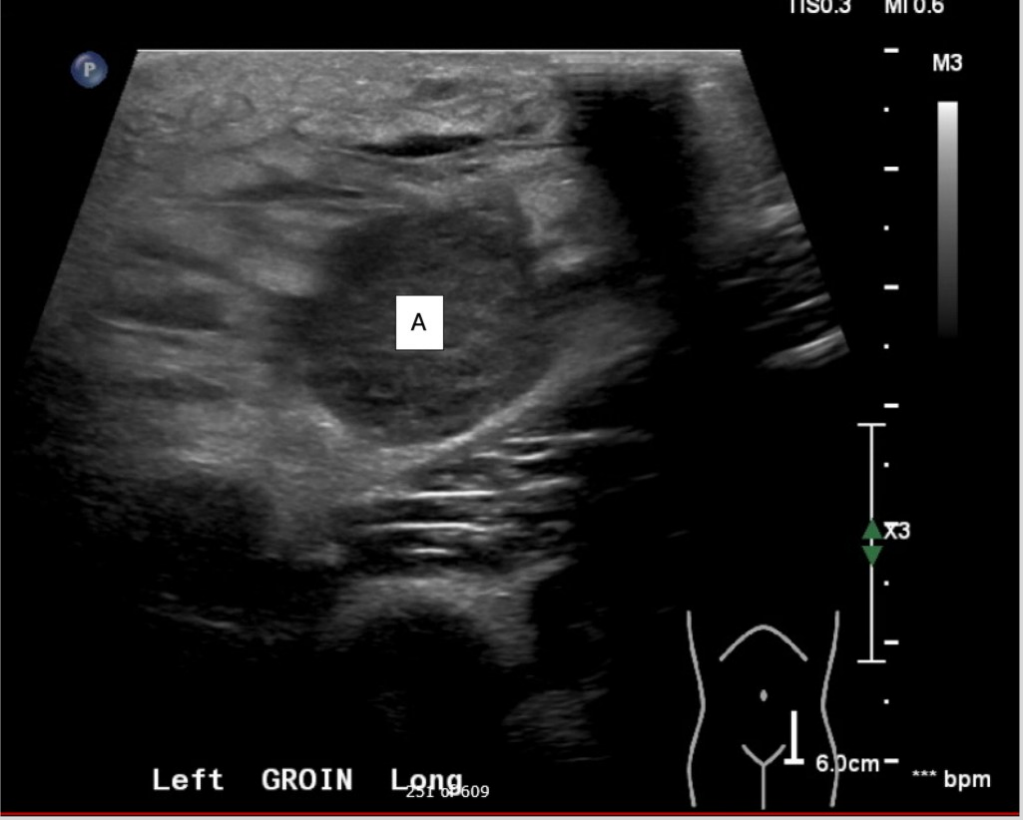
Radiology performed ultrasound of left groin during readmission demonstrating a heterogeneous peri-lymphatic fluid collection (A).
